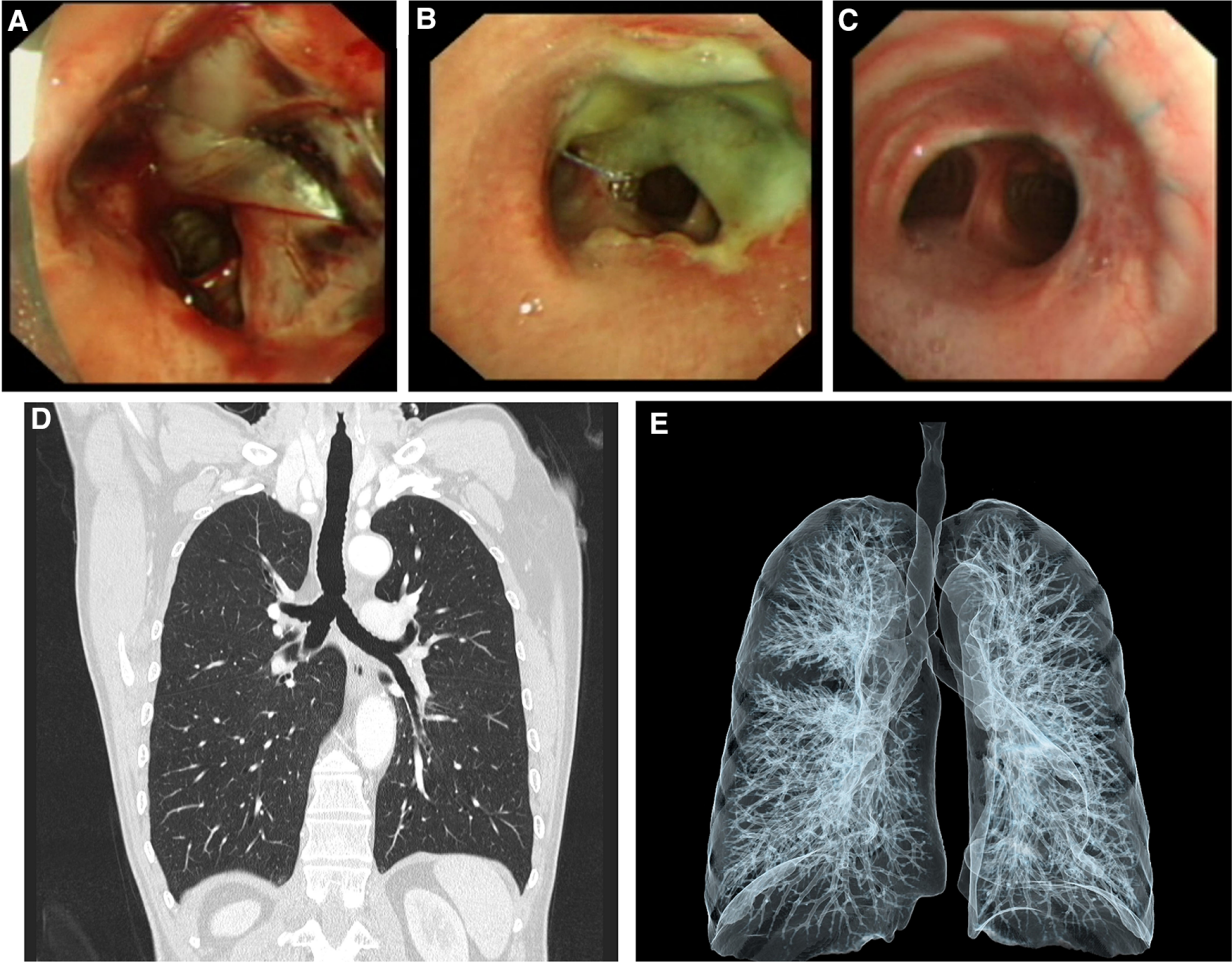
Fiberoptic bronchoscopy on the day of surgery (A), 10 days after surgery (B), and 6 months later (C). Fiberoptic bronchoscopy 6 months later showing a smooth mucosal on the inner surface of the repaired trachea. (D,E) Postoperative CT scan and 3D tracheal imaging 3 years later showing no tracheal stenosis or obstruction.
